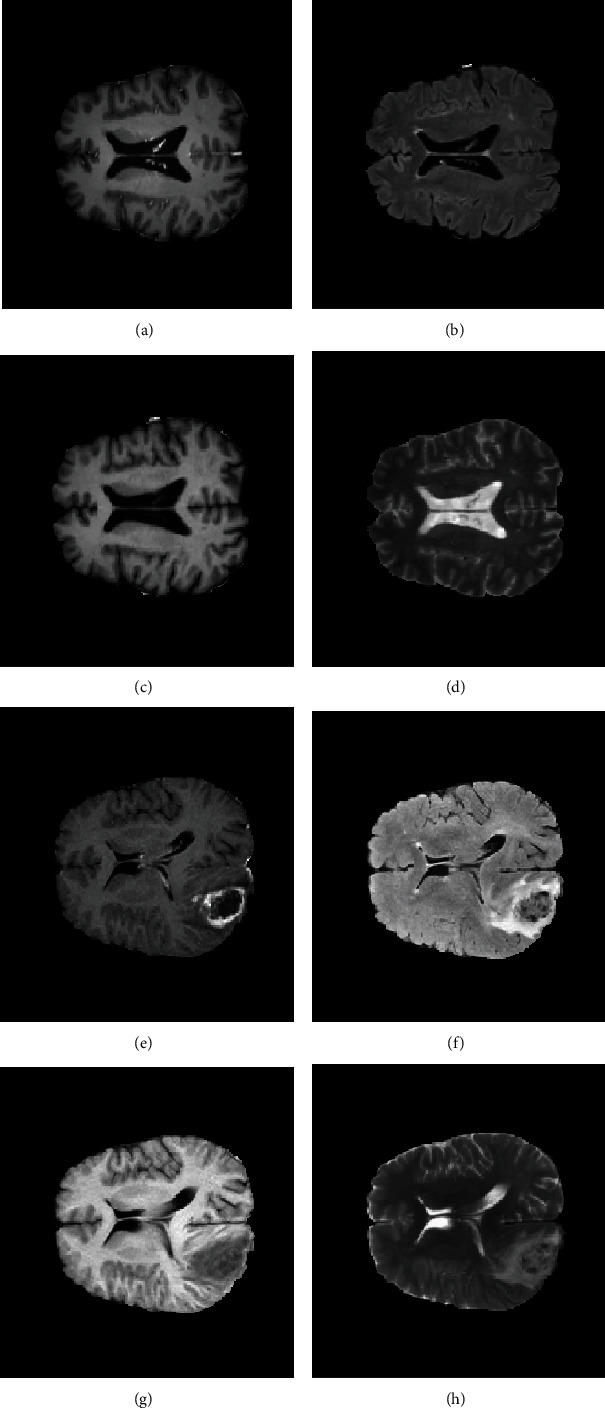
Samples from dataset for each class of brain tumors: (a) T1Ce benign image, (b) FLAIR benign image, (c) T1 benign image, (d) T2 benign image, (e) T1Ce malignant image, (f) FLAIR malignant image, (g) T1 malignant image, and (h) T2 malignant image.
